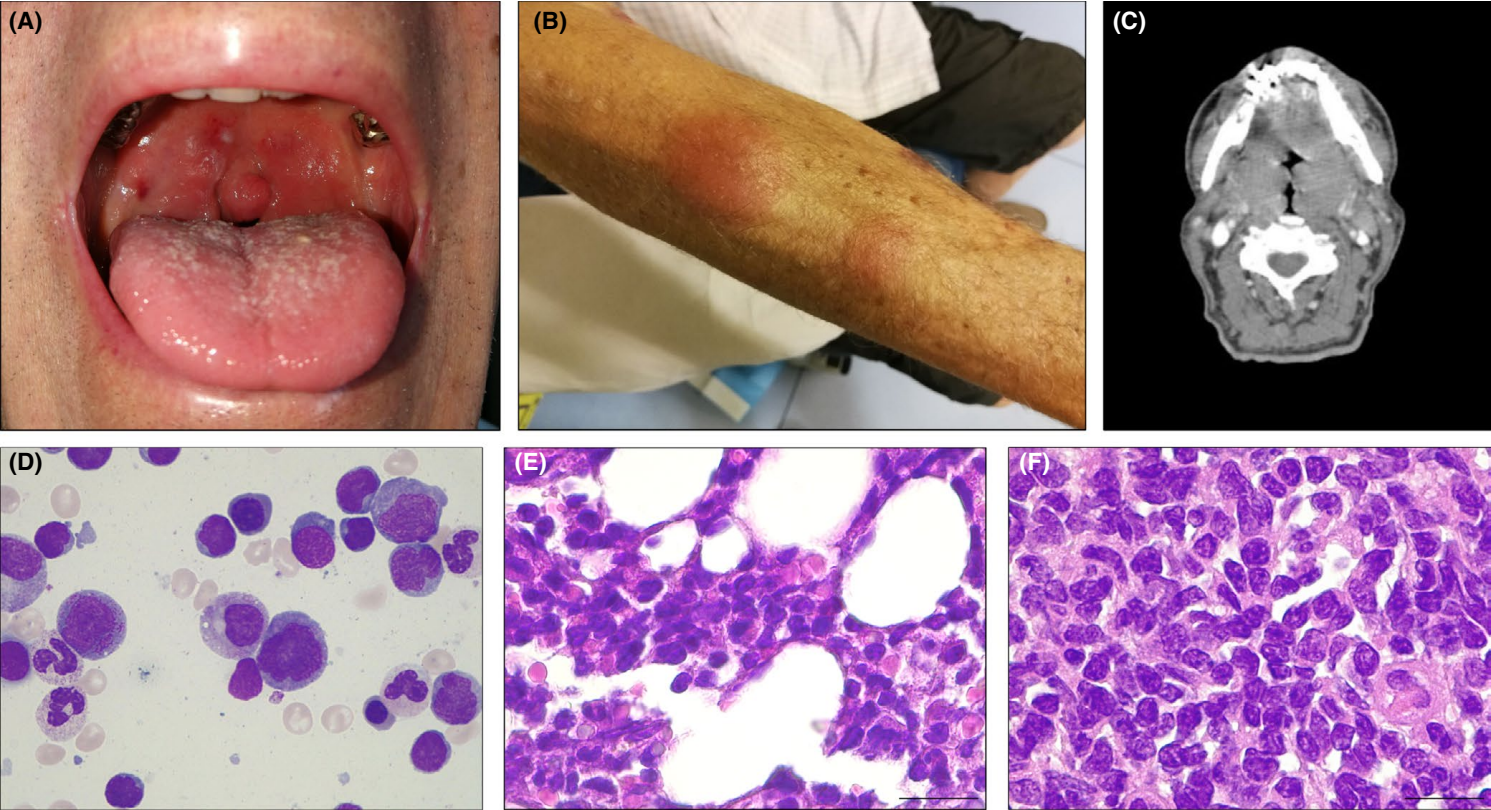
A, Bilateral tonsillar enlargement secondary to T‐cell prolymphocytic leukemia cells (T‐PLL) infiltration. B, Nodular skin infiltration. C, Axial contrast‐enhanced CT scan shows bilateral palatine tonsils enlargement. D, Bone marrow (BM) aspirate morphology showing T‐PLL cells. E, BM biopsy shows an abnormal lymphocytic infiltrate. F, Epithelium of tonsil showing diffuse infiltration of T‐PLL
